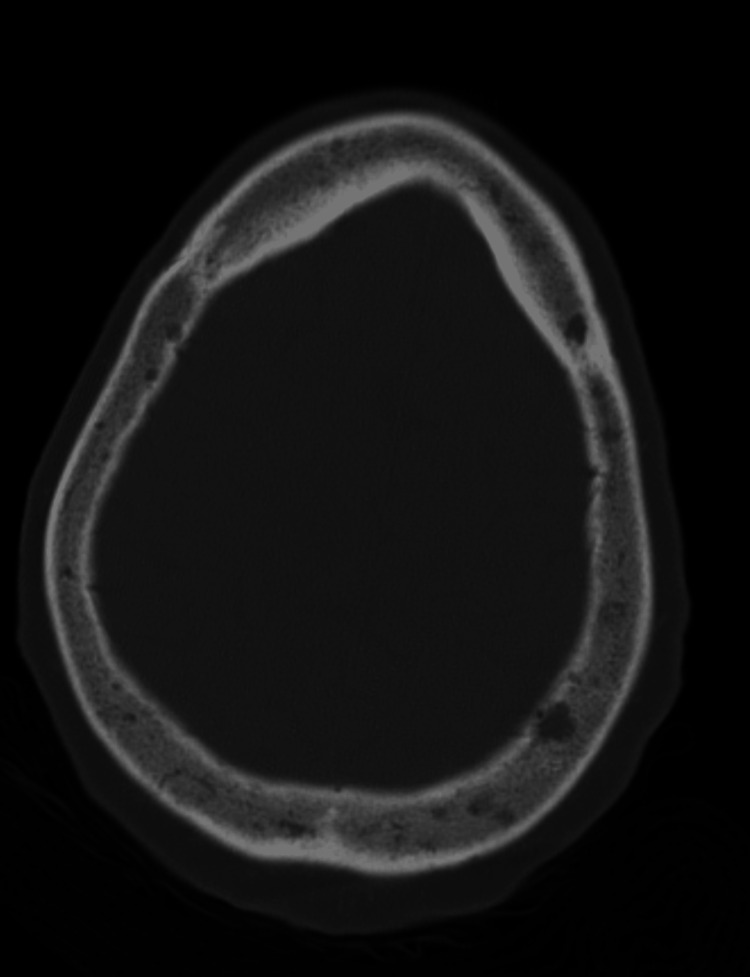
CT of the head demonstrating lytic lesions CT: computed tomography
